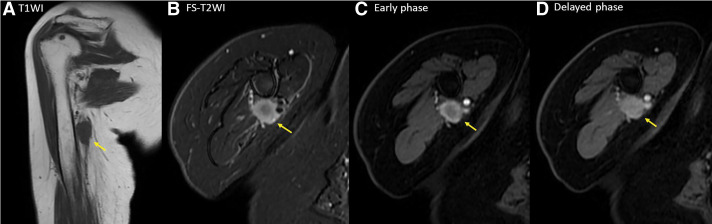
(A, B) MRI showing a well-defined mass with low signal intensity on T1-weighted imaging (coronal) and high signal intensity on fat-suppressed T2-weighted imaging (axial). (C, D) Contrast-enhanced MRI scan demonstrating strong contrast enhancement with rim predominance. T1WI, T1-weighted imaging; T2WI, T2-weighted imaging
